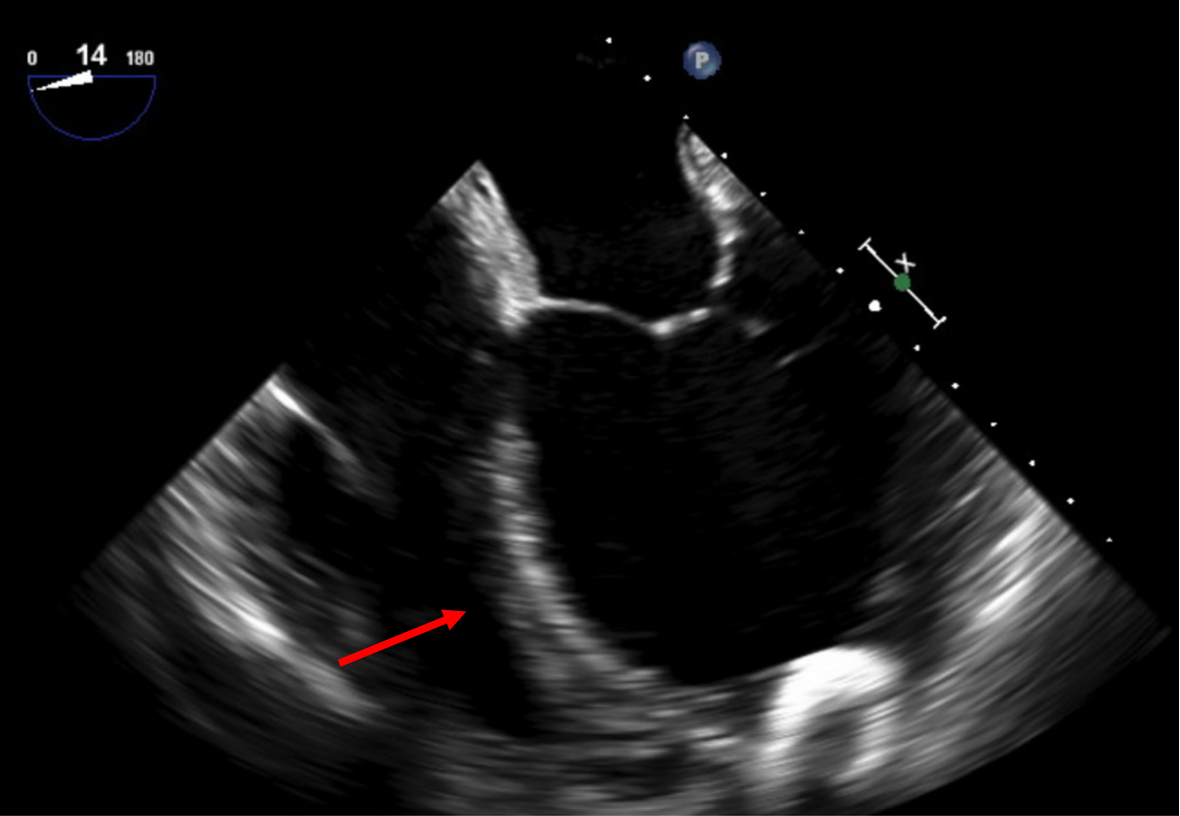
Transesophageal echocardiogram image showing bowing of the intraventricular septum bulging to the right during diastole. Arrow indicates intraventricular septum bowing
